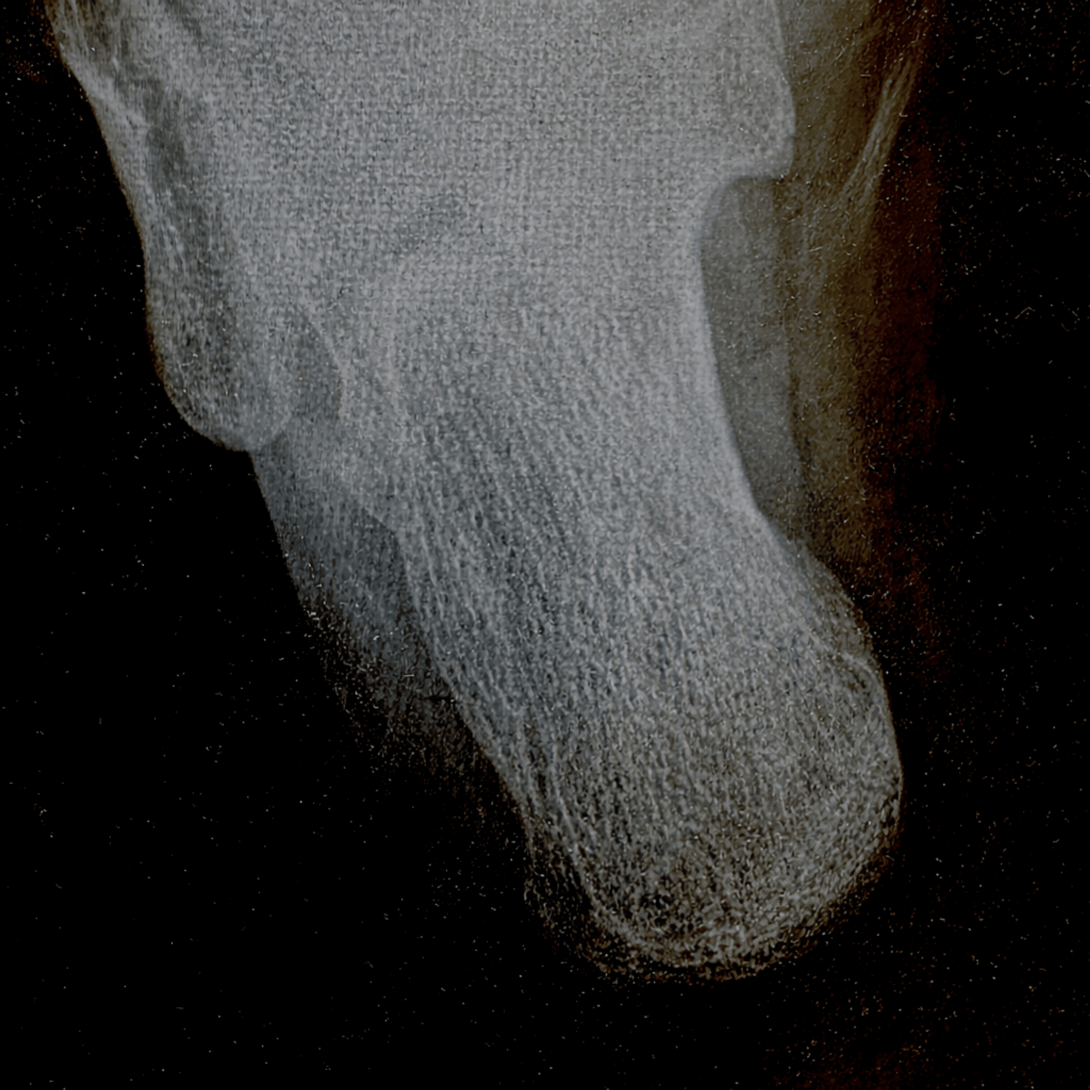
Post-removal radiograph of the foot. Post-procedural lateral radiograph demonstrating complete removal of the metallic wood screw, with no evidence of retained foreign body fragments or osseous injury.
